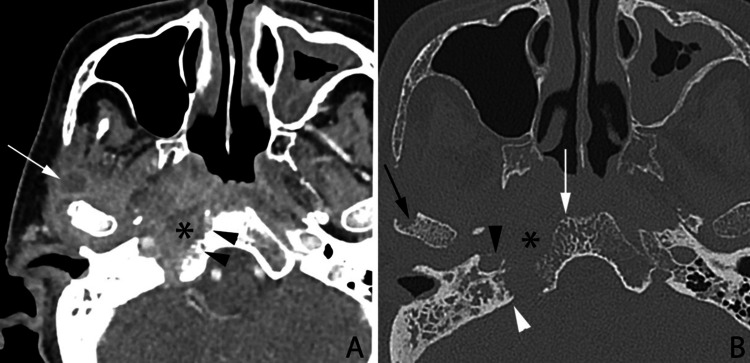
Axial contrast CT of the base of the skull in soft tissue (A) and bone algorithm (B) windows. Diffuse heterogeneously enhancing soft tissue with ill-defined margins is detected at the right temporomandibular joint, masticator, parapharyngeal, prevertebral spaces, and right nasopharynx, with suspicious encroachment upon the right jugular fossa. There is some suggestion of hypodensity within the right preclival region (asterisk in A), possibly due to early liquefaction. A discrete rim-enhancing ovoid lesion with fluid attenuation content centrally at the region of the right masseter indicates an abscess (white arrow in A). Superiorly, the extension of the said soft tissue enhancement is identified at the right foramen lacerum, with an erosion of the adjacent right carotid canal (black arrowhead in B), and cortical destruction at the right aspect of adjacent basisphenoid (black arrowheads in A). Bone algorithm CT displays lytic change within the right aspect of the basisphenoid marrow (white arrow in B), as well as erosion at the right jugular fossa (white arrowhead in B), occipital condyle, and hypoglossal canal (not shown). Note the eroded right petrous apex (asterisk in B, compared to the normal left counterpart). The rest of the right sigmoid sinus opacified unremarkably.
